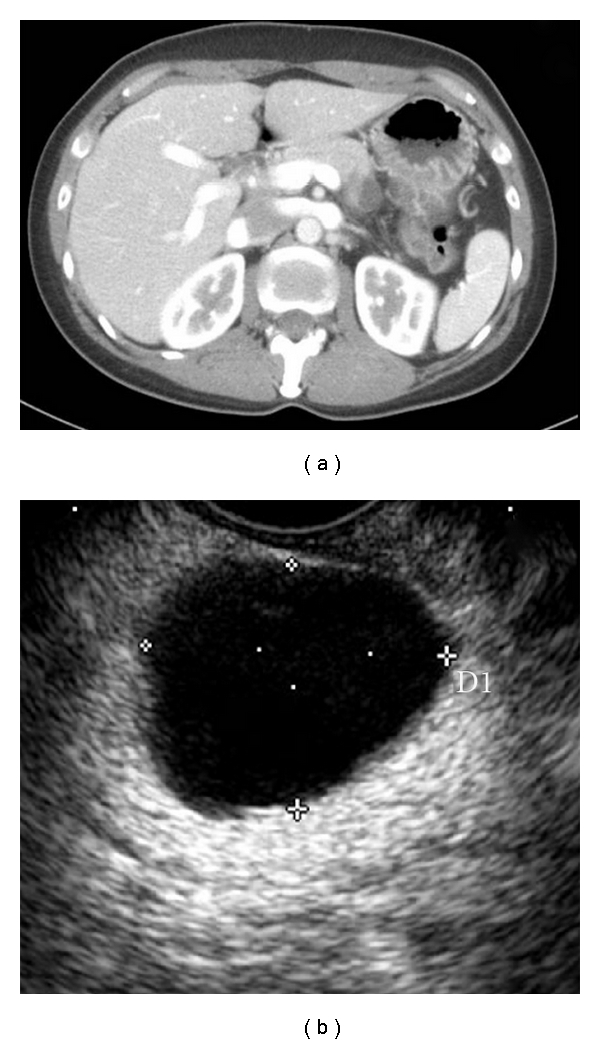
Mucinous cystic neoplasm. (a) Typical CT and (b) EUS appearance of a well-rounded hypodense and anechoic, respectively, pancreatic cyst in the tail of the gland of a female patient.
